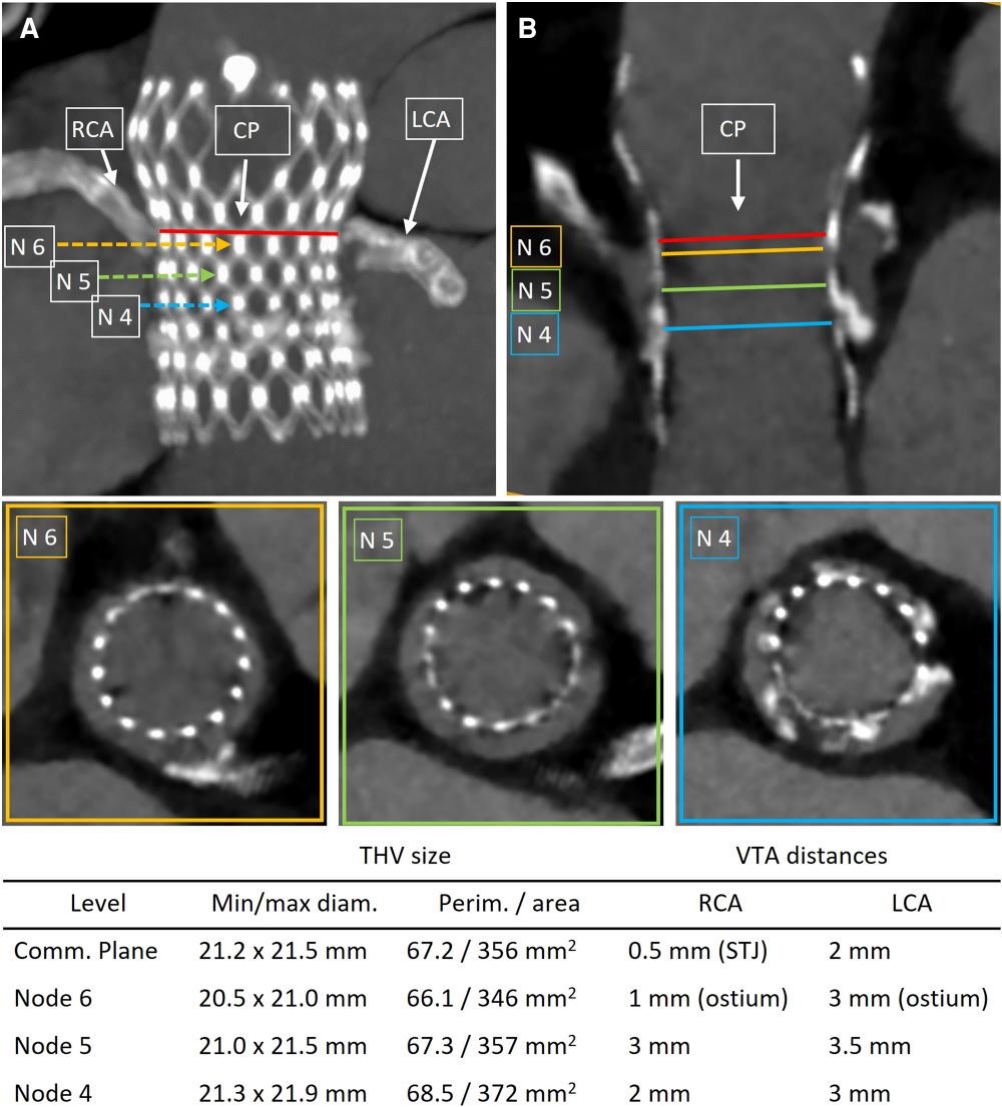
Step-by-step computed tomography analysis of index transcatheter aortic valve replacement. Identification of Evolut transcatheter heart valve commissural plane (red line), located above the coronary ostia, and identification of Evolut transcatheter heart valve node 6, 5, and 4 planes in a maximum intensity projection (*A*) and a longitudinal view (*B*). Axial view at Evolut transcatheter heart valve node 6 (N6), showing a prohibitive valve-to-aorta distance to right coronary artery. Axial views at Evolut node 5 (N5) and node 4 (N4), displaying more permissive valve-to-aorta distances. The table below summarizes Evolut transcatheter heart valve size and valve-to-aorta distances at each level. CP, commissural plane; CT, computed tomography; LCA, left coronary artery; RCA, right coronary artery; N6, node 6; N5, node 5; N4, node 4; VTA, valve-to-aorta; THV, transcatheter heart valve.
